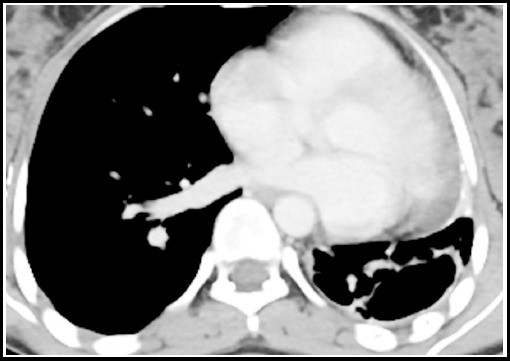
CT scan with IV contrast section shows pericardial thickening with calcification. Streak of pericardial effusion is also seen.
